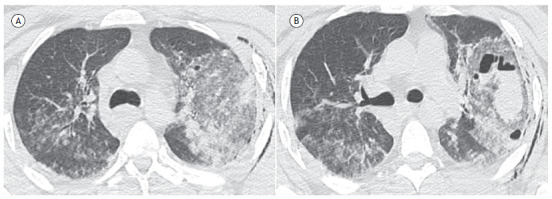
Axial CT scans with lung window settings showing extensive ground-glass opacities in the left lung (pulmonary contusion), interspersed with oval images with air and fluid content, forming air-fluid levels (pulmonary lacerations). Also note soft tissue emphysema and discrete ground-glass opacities on the right.
